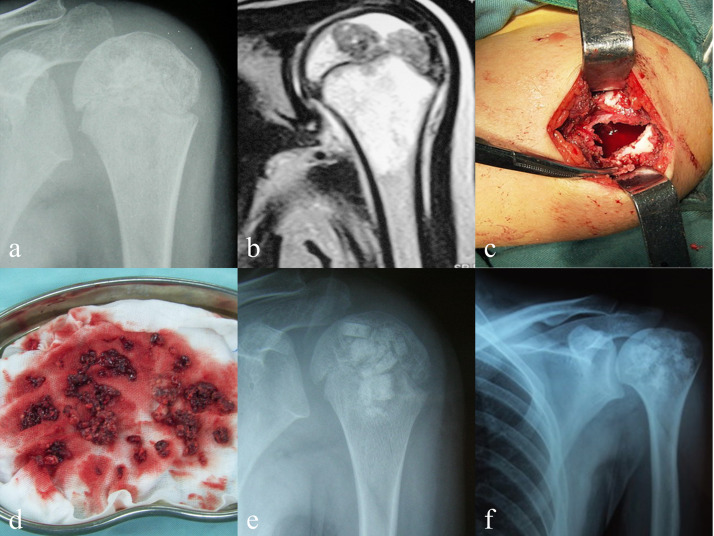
An 11-year-old boy with chondroblastoma involving the proximal humerus of the left side treated with intralesional curettage, electrocauterization of the cavity and bone grafting. a, b Radiographs showing eccentric round-like focus located in the epiphysis with a lytic lesion on X-ray film and Magnetic Resonance Imaging scan. c Intraoperative photo after curettage (the proximal humerus). d Gross appearance of pathological tissues. e Postoperative radiograph showing the lesions after curettage and grafting. f Radiograph at the 8-year follow-up.
